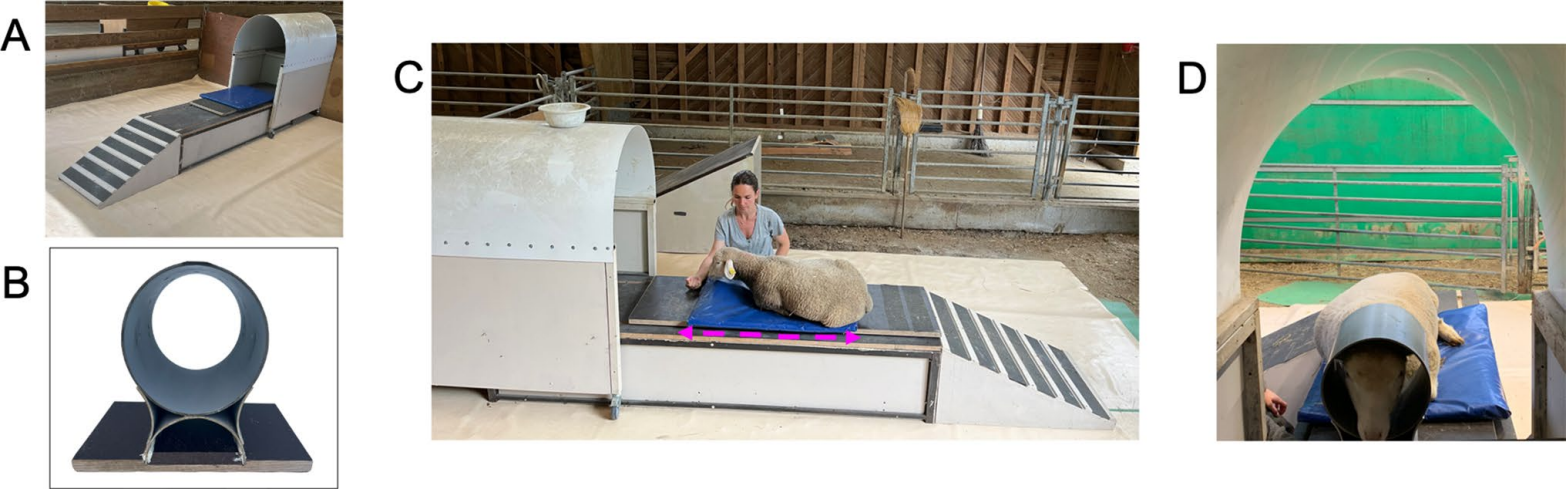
Learning MRI acquisition-related behaviors. A The mock MRI scanner composed of a ramp, a fixed base on which a mock patient bed sits and a mock bore; B The mock RF head coil; C Ted lying down on the moveable mock MRI table (*dashed pink arrow*), on a comfortable blue mat, in front of the mock MRI bore; D Léonard waiting motionless with his head inside the mock RF head coil
